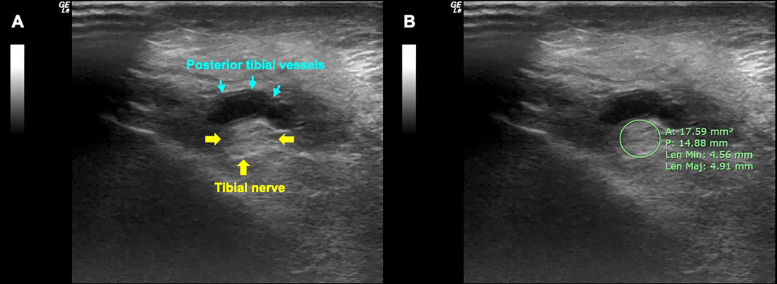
High-frequency ultrasound at the ankle showing the tibial nerve (A) and measurement of its cross-sectional area (B). Yellow arrowheads indicate the posterior tibial vessels and the red arrows indicate the boundaries of the tibial nerve.
